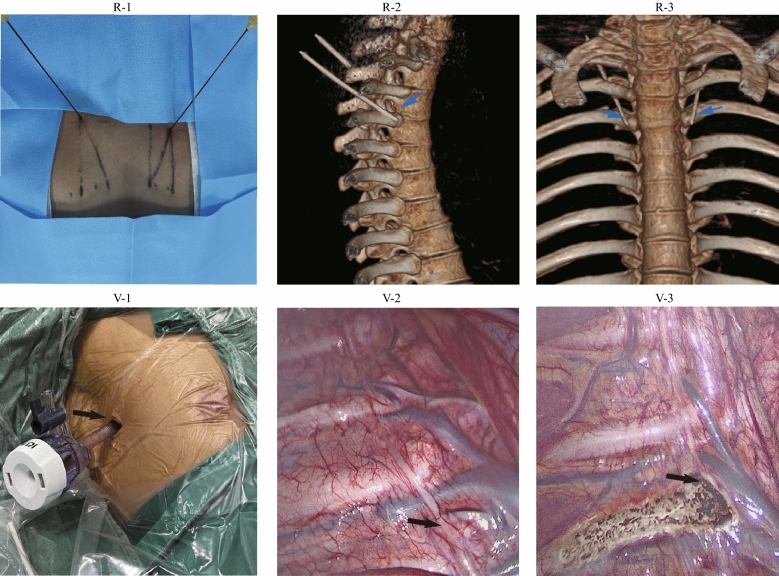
Radiofrequency ablation (RFA) and video-assisted thoracoscopic sympathectomy (VATS) for treatment, with pathways and procedures at the fourth thoracic level. R-1: Body surface for the operative approach in RFA. R-2: Lateral film for 3-dimensional X-ray computed tomography imaging in RFA. R-3: Positive film for 3-dimensional X-ray computed tomography imaging in RFA. V-1: Body surface for the operative approach in VATS (third/fourth intercostal space). V-2: Straight view of the sympathetic nerve before surgery with VATS. V-3: Straight view of the sympathetic nerve after surgery with VATS.
